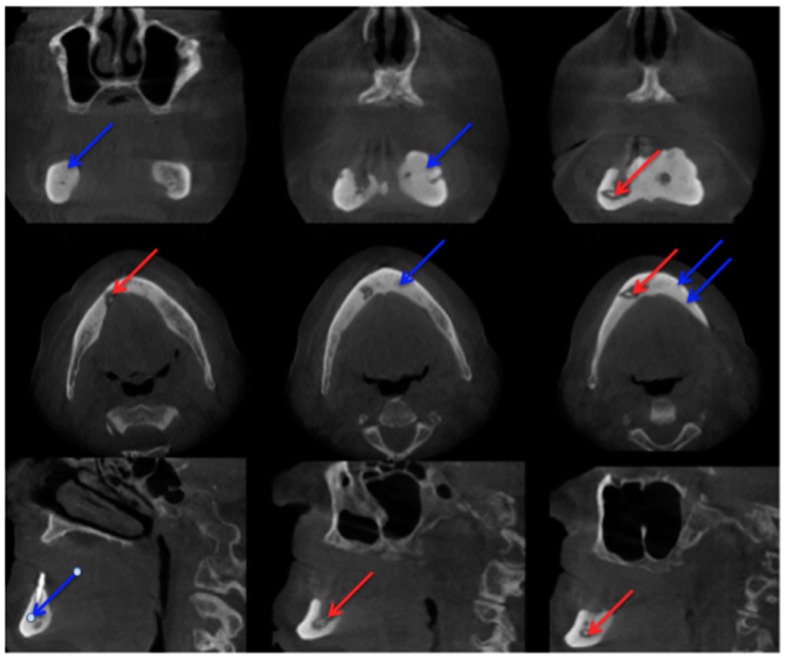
Cone-beam computed tomography (Carestream CS 9300) Patient: 77 years old, metastatic prostate cancer, ibandronic acid and later another antiresorptive drug: denosumab. For panoramic radiograph see Figure 2. 1st row: coronary view; 2nd row: axial view; and 3rd row: sagittal view. Red arrows: sequester; blue arrows: sclerotic region. AAMOS staging: stage 2.
